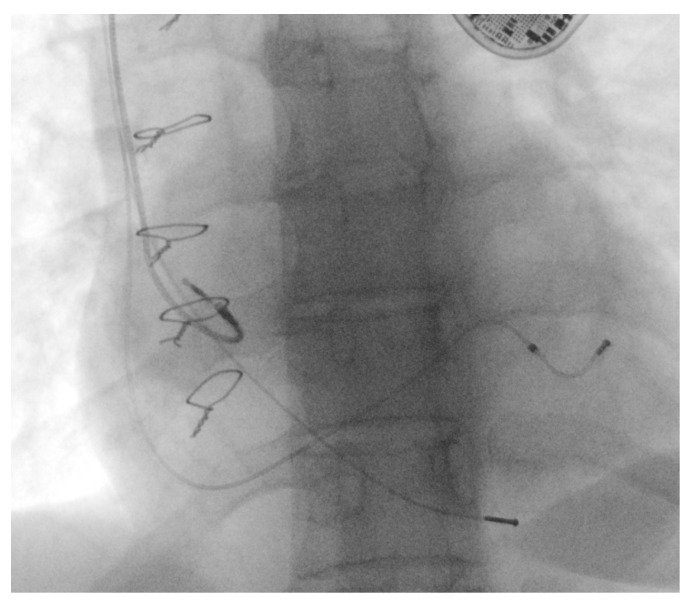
Chest X-ray at the time of admission at the hospital, with one lead positioned in the right atrial appendage, one on the apex of the right ventricle, and another one in the posterolateral vein.
